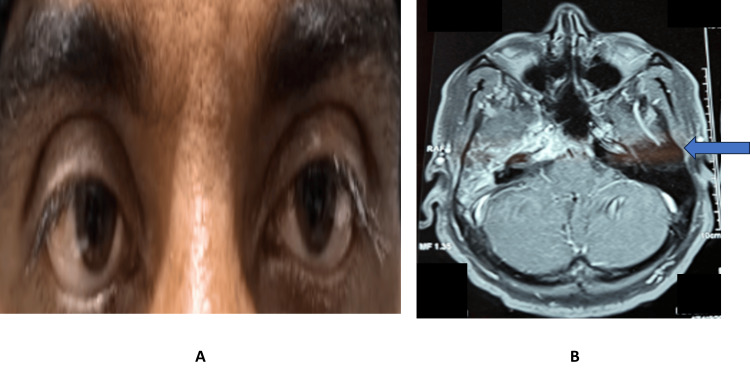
Clinical and imaging features of isolated lateral rectus palsy. (A) Right eye lateral rectus palsy. (B) CT scan showing petrositis image.
